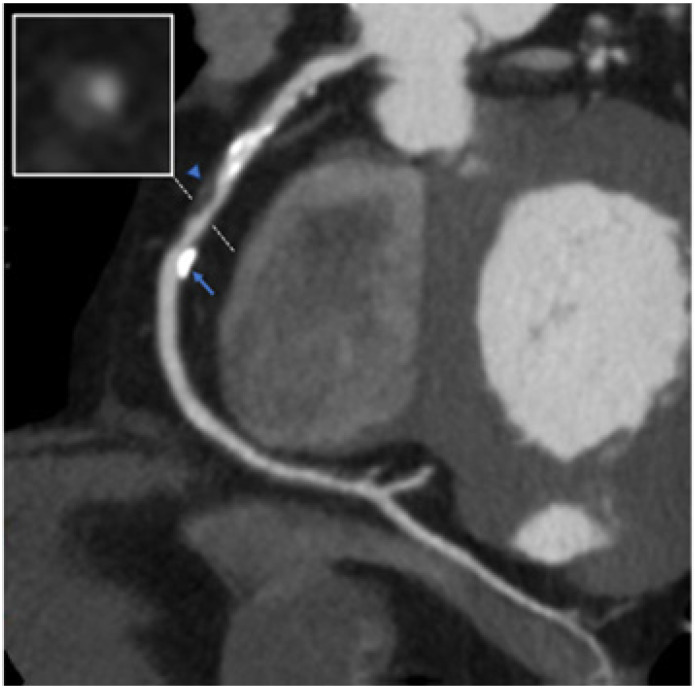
Coronary computed tomography angiography (CCTA) demonstrating 70–90% stenosis in the right coronary artery (RCA). A solid arrow indicates calcified plaque, while a blue triangular arrow highlights non-calcified plaque. A short-axis image (white dashed line) obtained using double oblique reconstruction shows plaque with high-risk features (napkin-ring sign).
